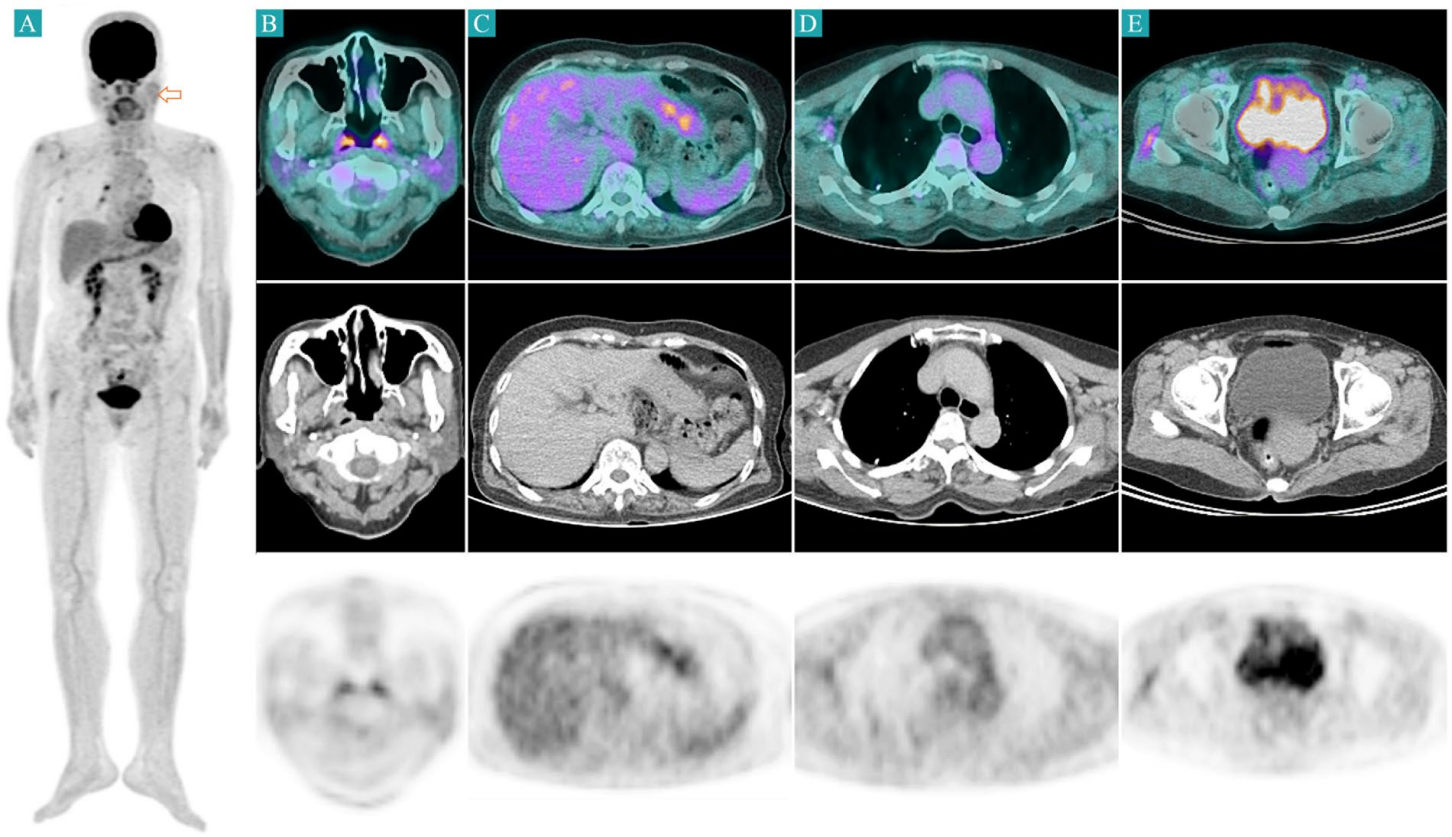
Follow-up FDG PET/CT image of the same patient in Fig. 3. FDG uptake and the size of eschar, lymph nodes, and spleen are markedly decreased after proper antibiotic therapy (A–E). The red empty arrow indicates the eschar lesion.
